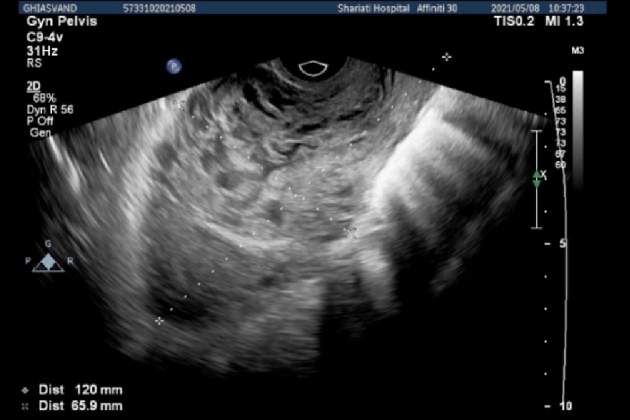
Transvaginal ultrasonography (uterine size = 65
